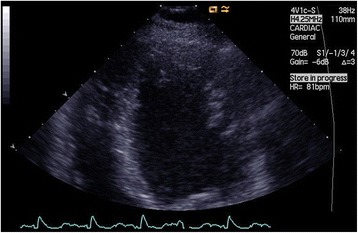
Transthoracic echocardiogram, end-diastole, apical four-chamber view showing prominent trabeculations in the left ventricular wall
